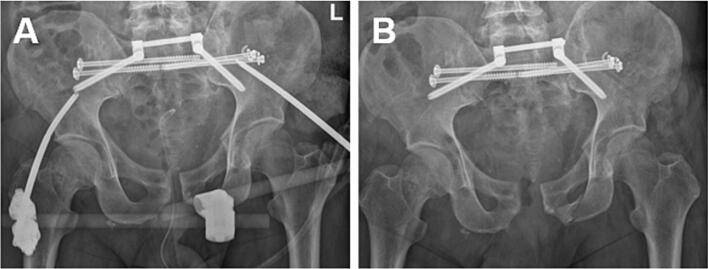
Postoperative anterior-posterior X-ray of the pelvis after posterior stabilization with bilateral sacroiliac screws and transiliac internal fixator and closed reduction and external fixation of the anterior pelvic ring (A). Persistent instability of the symphysis after removal of the external fixator eight weeks post-trauma (B).
